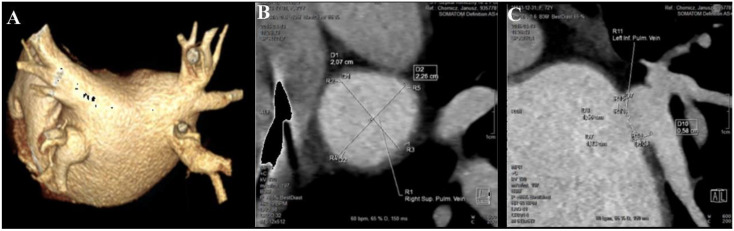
Pulmonary vein anatomy addressed by a 64-row-multidetector computed tomography. Three-dimensional left atrial reconstructed model in the postero-anterior view (panel A) and the example of pulmonary vein ostial maximum and minimum dimension analysis obtained from multiplanar reconstructed images using a dedicated caliper software: the right upper pulmonary vein in transverse cross-section (panel B) and the left inferior pulmonary vein in longitudinal cross-section (panel C).
